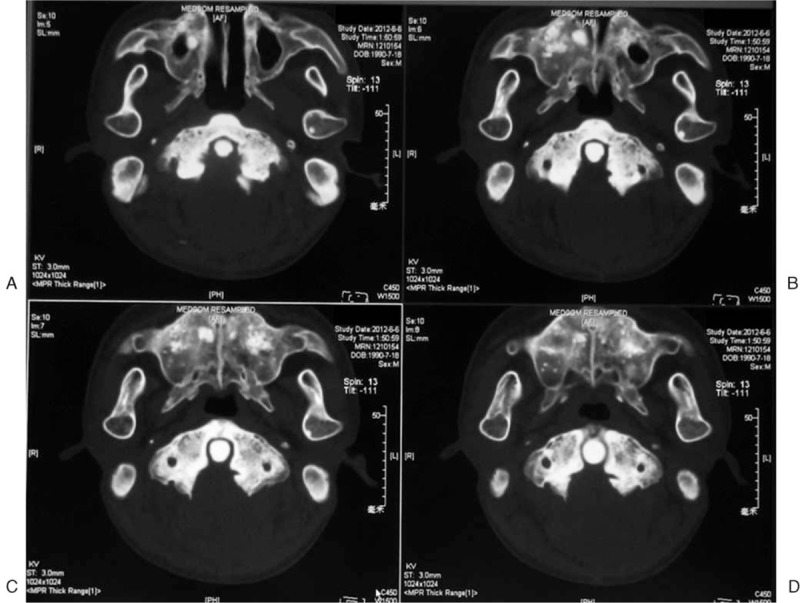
Continuous CT scans at the level of the atlas posterior arch. (A) The medial bone of the right VA groove was ground, whereas the left was preserved. (B–D) The bone groove ground during resection of the posterior ring was V-shaped, and the shallow grinding width was much larger than that deeper in the bone. VA = vertebral artery.
